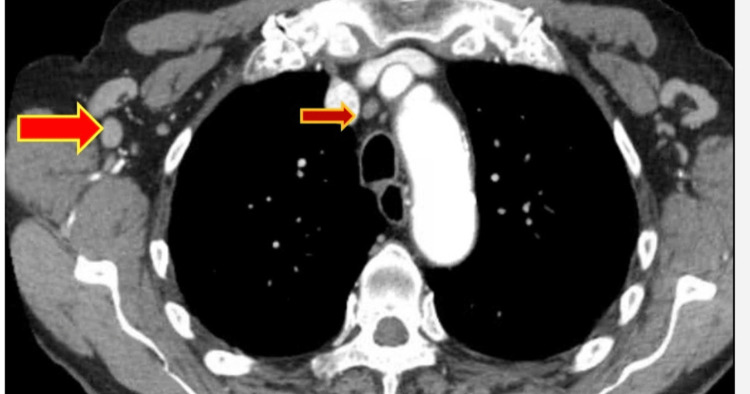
CT abdomen showing inguinal lymphadenopathy.
